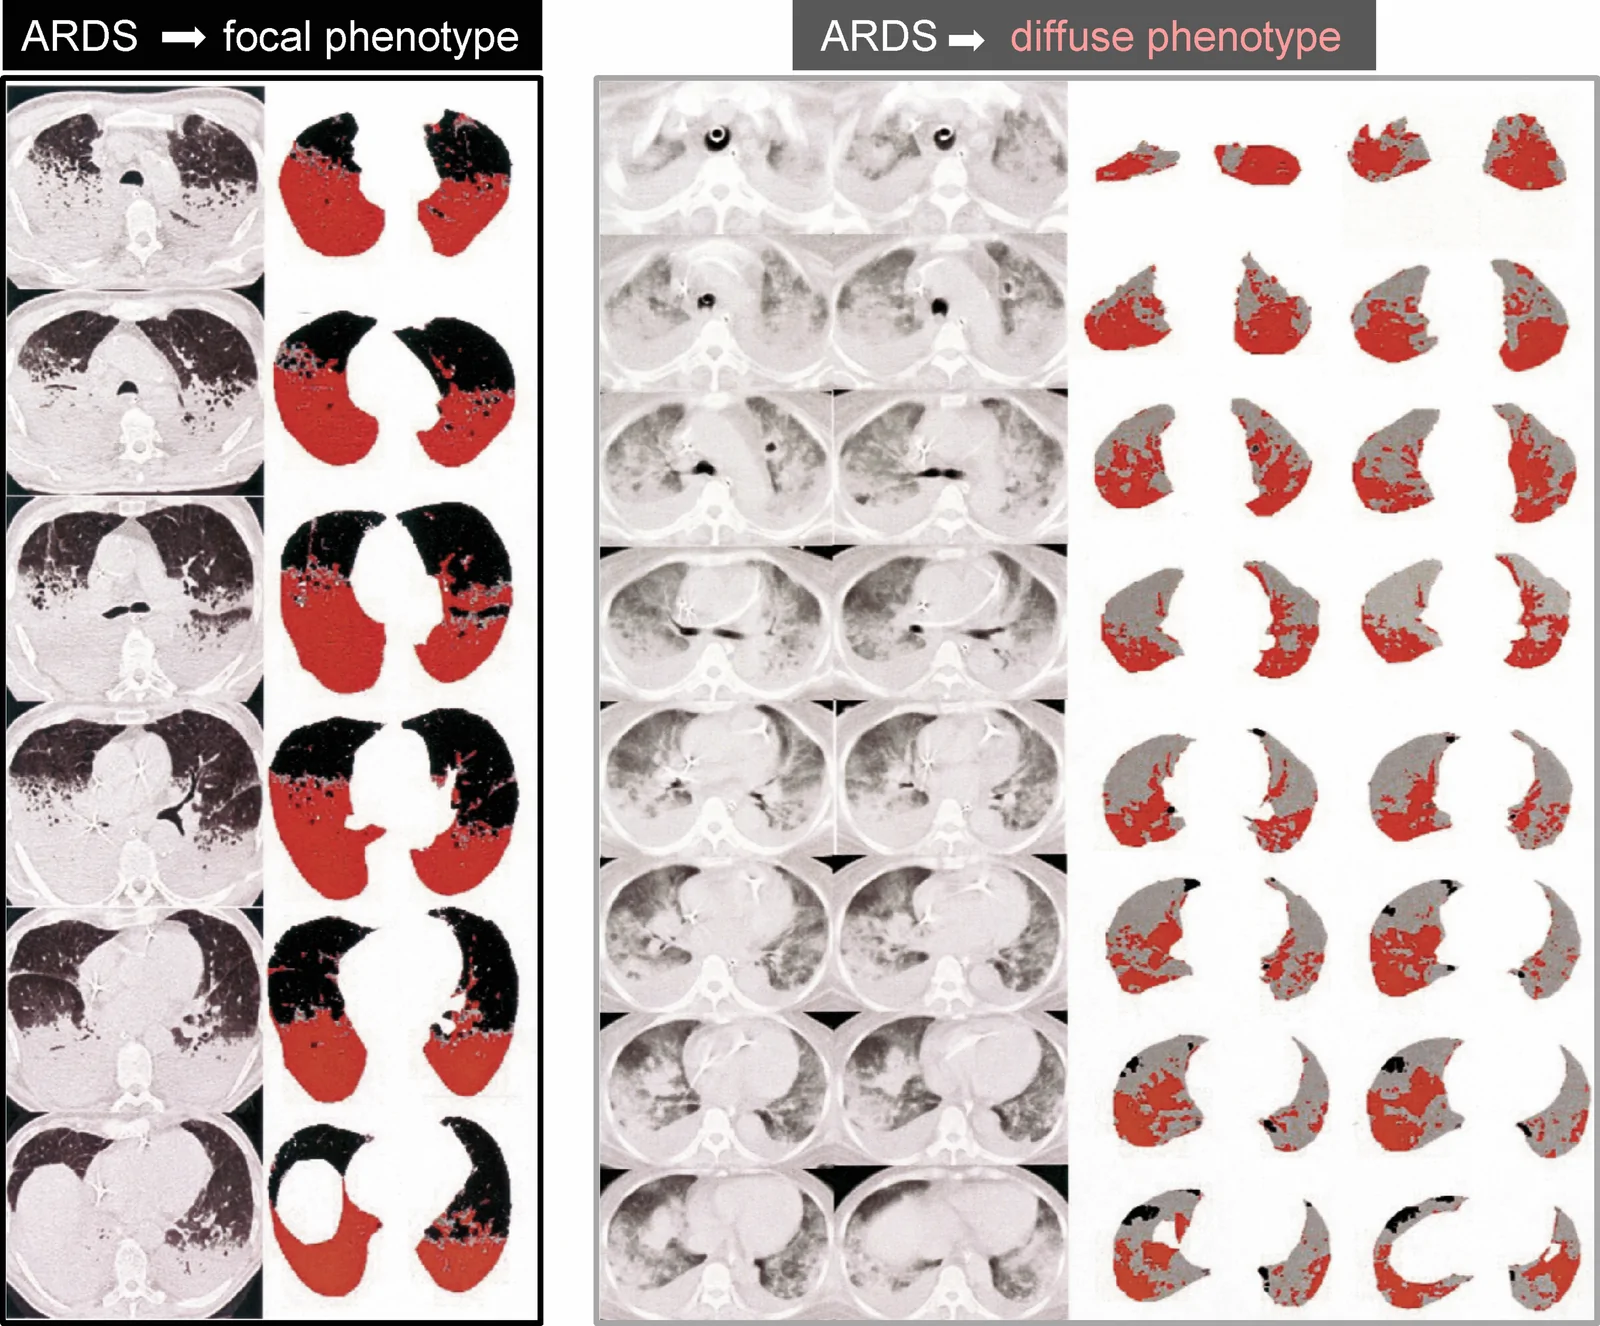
Acute Respiratory Distress Syndrome (ARDS) phenotypes based on the distribution of aeration loss (lung morphology). (A) Focal ARDS phenotype. Computed tomographic (CT) scan was performed in a 61 yr-old patient with ARDS caused by aspiration pneumonia in the postoperative period of a hip replacement. The color-coded CT sections show the distribution of normal aeration in black [CT attenuations ranging between -900 and -500 Hounsfield units (HU)], partial loss of aeration in gray (CT attenuations ranging between -500 and -100 HU) and total loss of aeration in red (CT attenuations ranging between -100 and +100 HU). Dependent parts of upper, middle and lower lobes are non aerated whereas nondependent parts show normal aeration. The proportion of poorly aerated lung is small. (B) Diffuse ARDS phenotype. CT scan was obtained in a 50 yr-old patient with ARDS caused by *Pneumocystis carinii*. Poorly and non aerated lung regions are predominant and homogeneously distributed. The proportion of normally aerated lung is small and essentially present in nondependent parts of the right middle lobe. Reproduced from reference^13^ with permission of the publisher.
